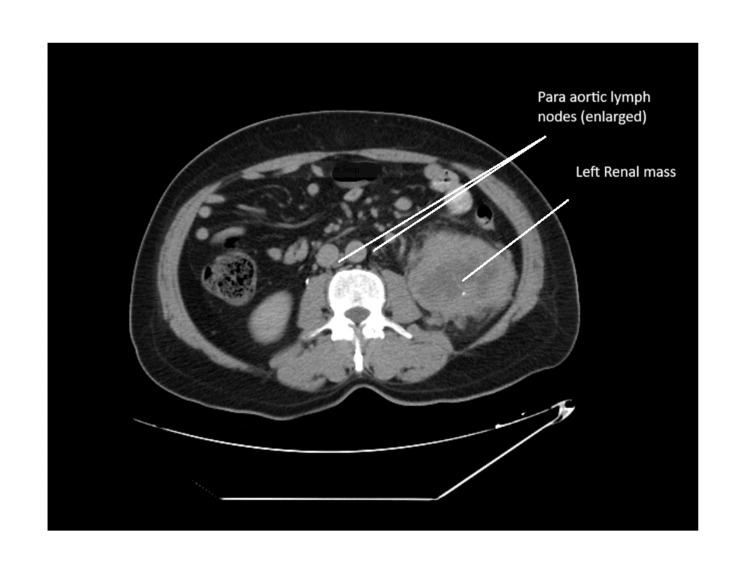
A CT scan of the abdomen A CT scan of the abdomen revealed a left renal mass measuring 10.1 x 7.9 x 13.7 cm, accompanied by enlarged para-aortic lymph nodes measuring 2.4 x 1.8 cm. CT, computed tomography
